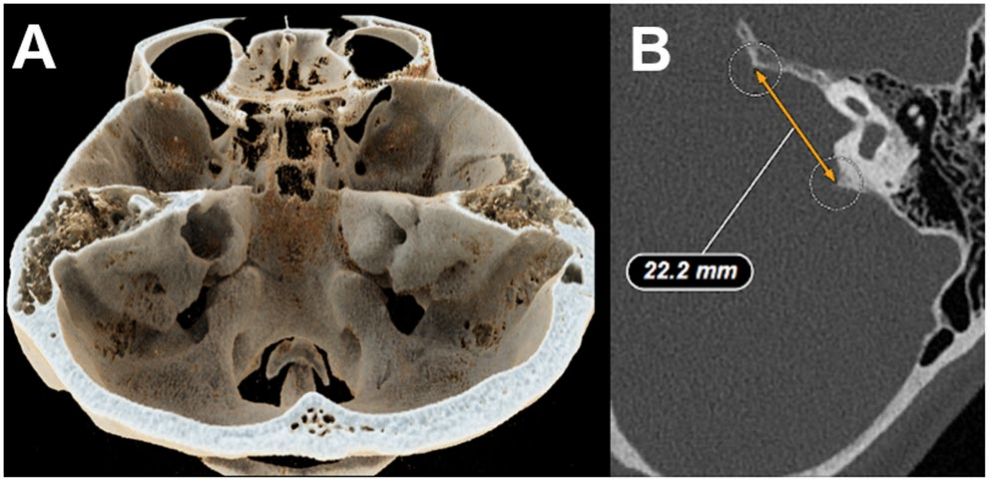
Panel A shows a three-dimensional reconstruction of the widened left IAC and panel B the measurement in the axial preoperative CT-scan
